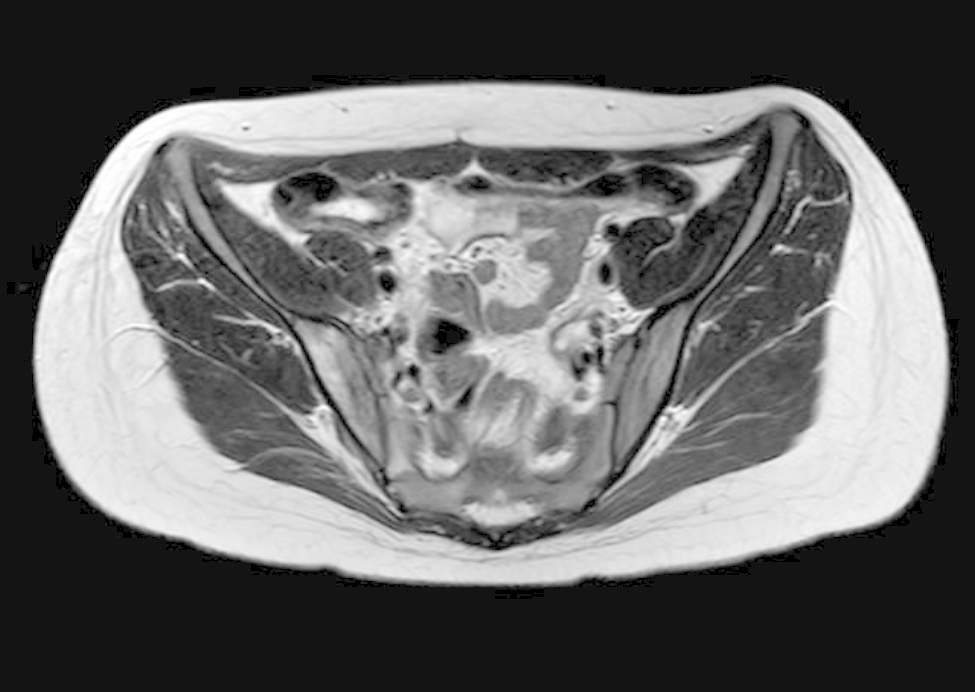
Magnetic Resonance Imaging after two months. we observed a completely resolution of muscles edema and abscess formation
